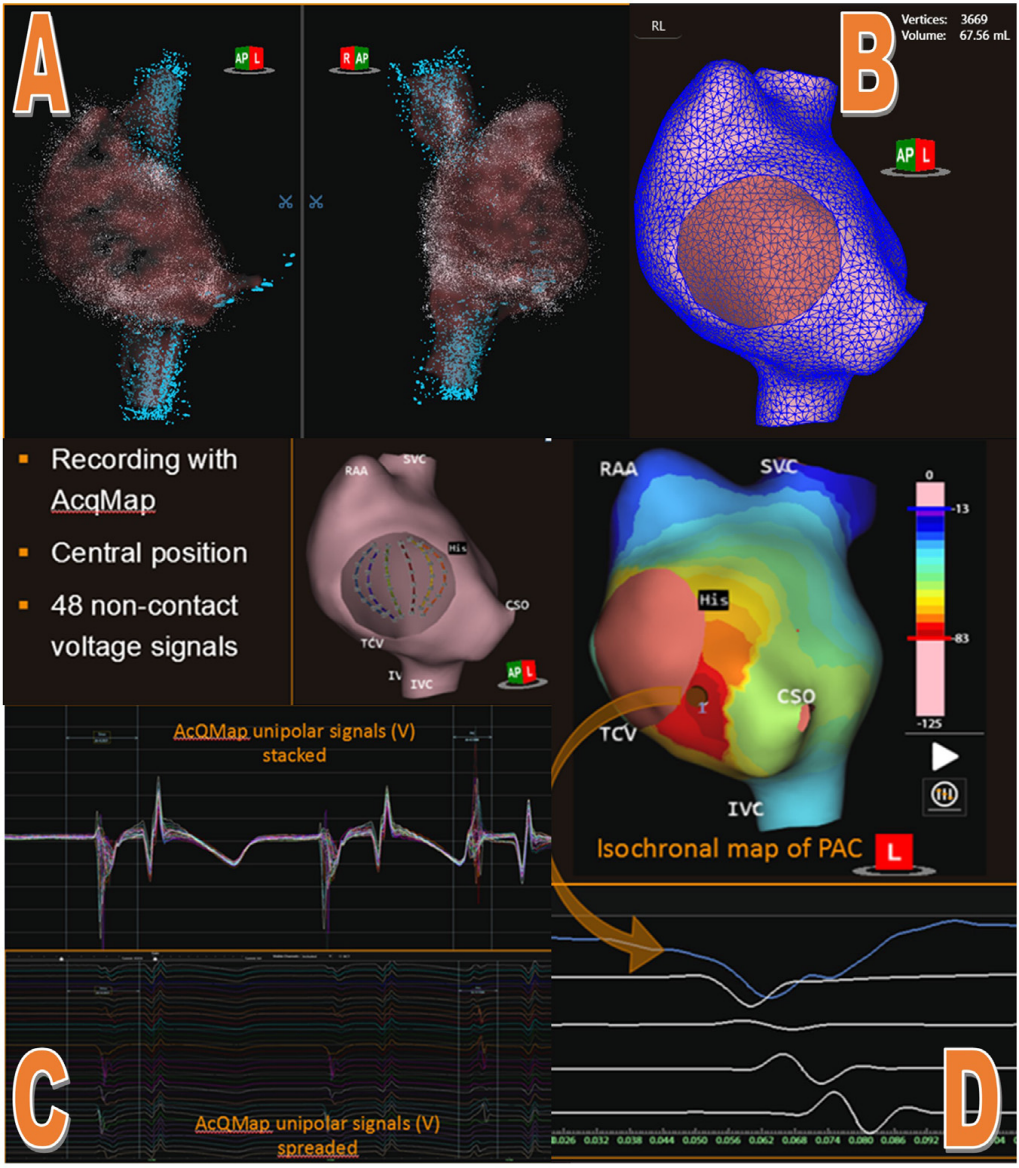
Creation of the right atrial activation map (AcQMap; Acutus Medical, Carlsbad, CA). A: Creation of the ultrasound point cloud. B: The resulting mesh network. C: Acquisition of signals. D: The resulting activation map together with the local signals at the site of earliest activation (*blue line and orange arrow*). CSO = coronary sinus ostium; IVC = inferior vena cava; PAC = premature atrial complex; RAA = right atrial appendage; SVC = superior vena cava; TCV = tricuspid valve.
